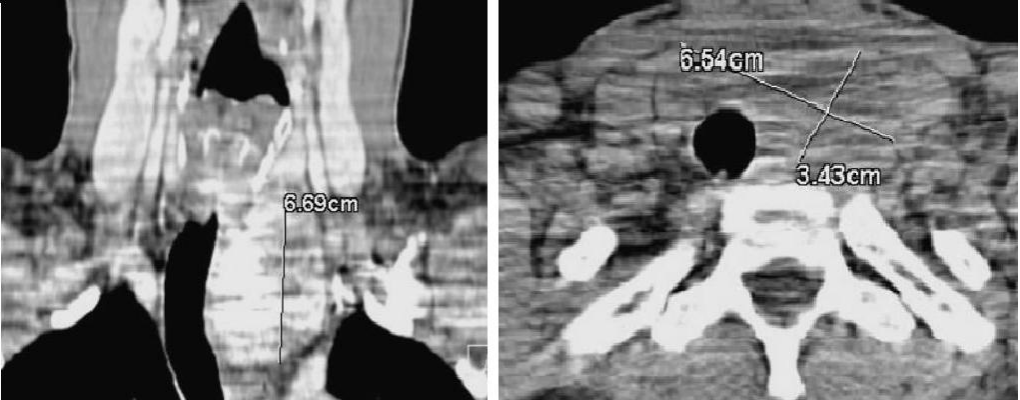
CT of the neck and chest confirming a posterior mediastinal mass arising from the left thyroid lobe at level of the thoracic outlet.
